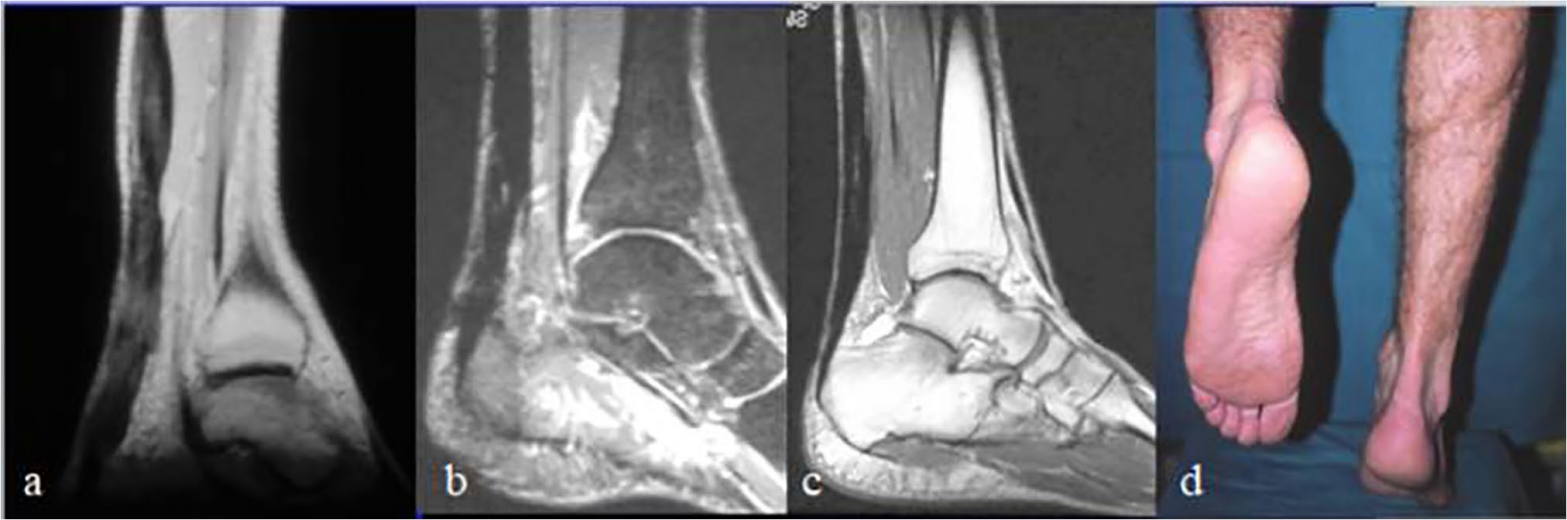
a Magnetic resonance imaging scan of male patients 34 years old 3 months postoperatively with satisfactory healing. b Magnetic resonance imaging scan 6 months postoperatively. c Magnetic resonance imaging scan at final follow-up. d Single heel rise 3 months postoperatively
